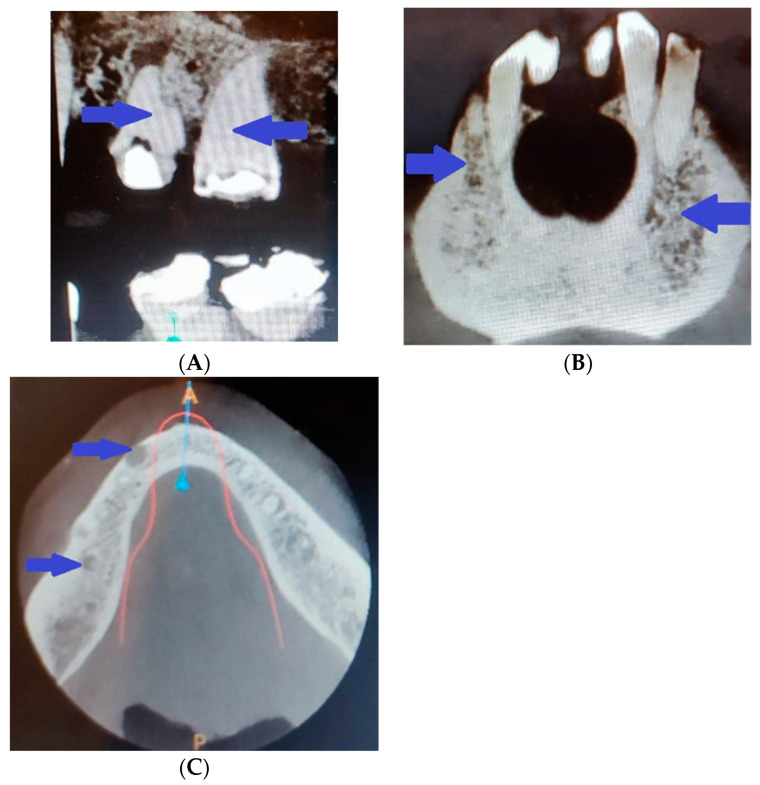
Radiography revealing (A) pulp calcifications and obliteration of the pulp cavity; (B) spongy bone structure; (C) some cystic masses.
